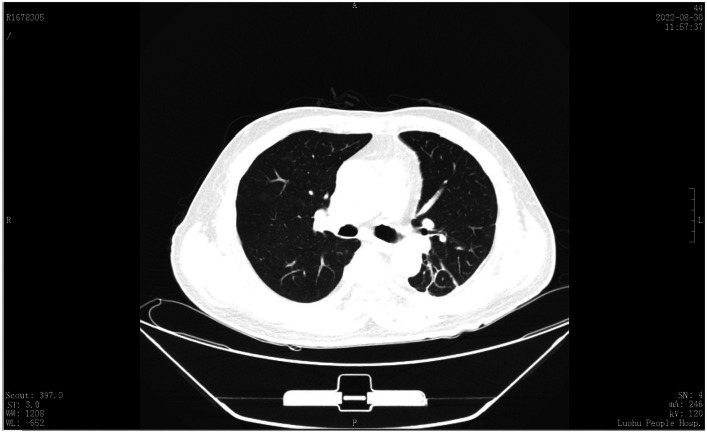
Recheck chest CT was showed pleural effusion disappeared 21 days after the operation.
